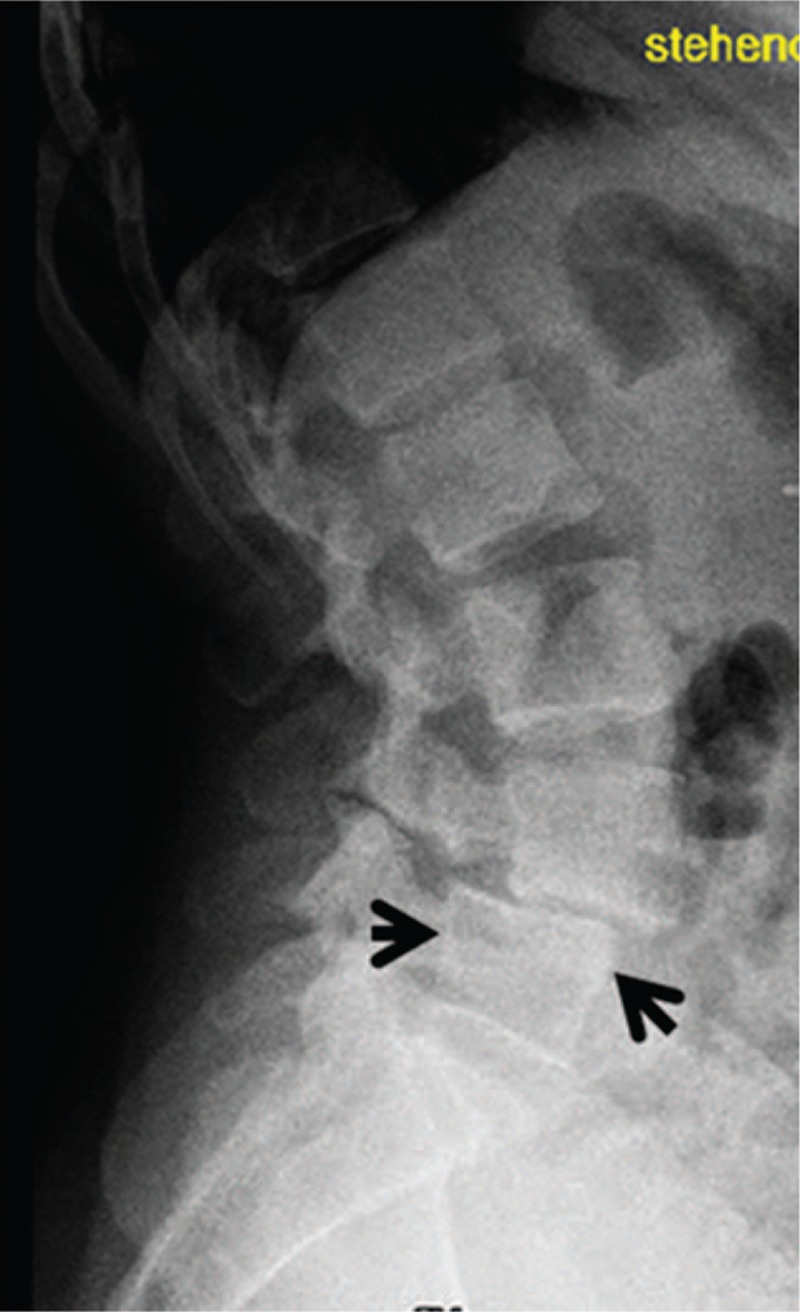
Lateral spine radiograph of the mother (III,6) showed a bilateral break in the pars interarticularis (lucency shown by arrow head). The latter was the reason to develop forward slippage of L5 on the S1; she is a heterozygous carrier of disease causing mutations in the *ARSB* gene as well as in the *GNS* gene, which denote an impaired lysosomal degradation of dermatan sulfate and heparan sulfate in the fully developed diseases MPS VI and MPS IIID.
